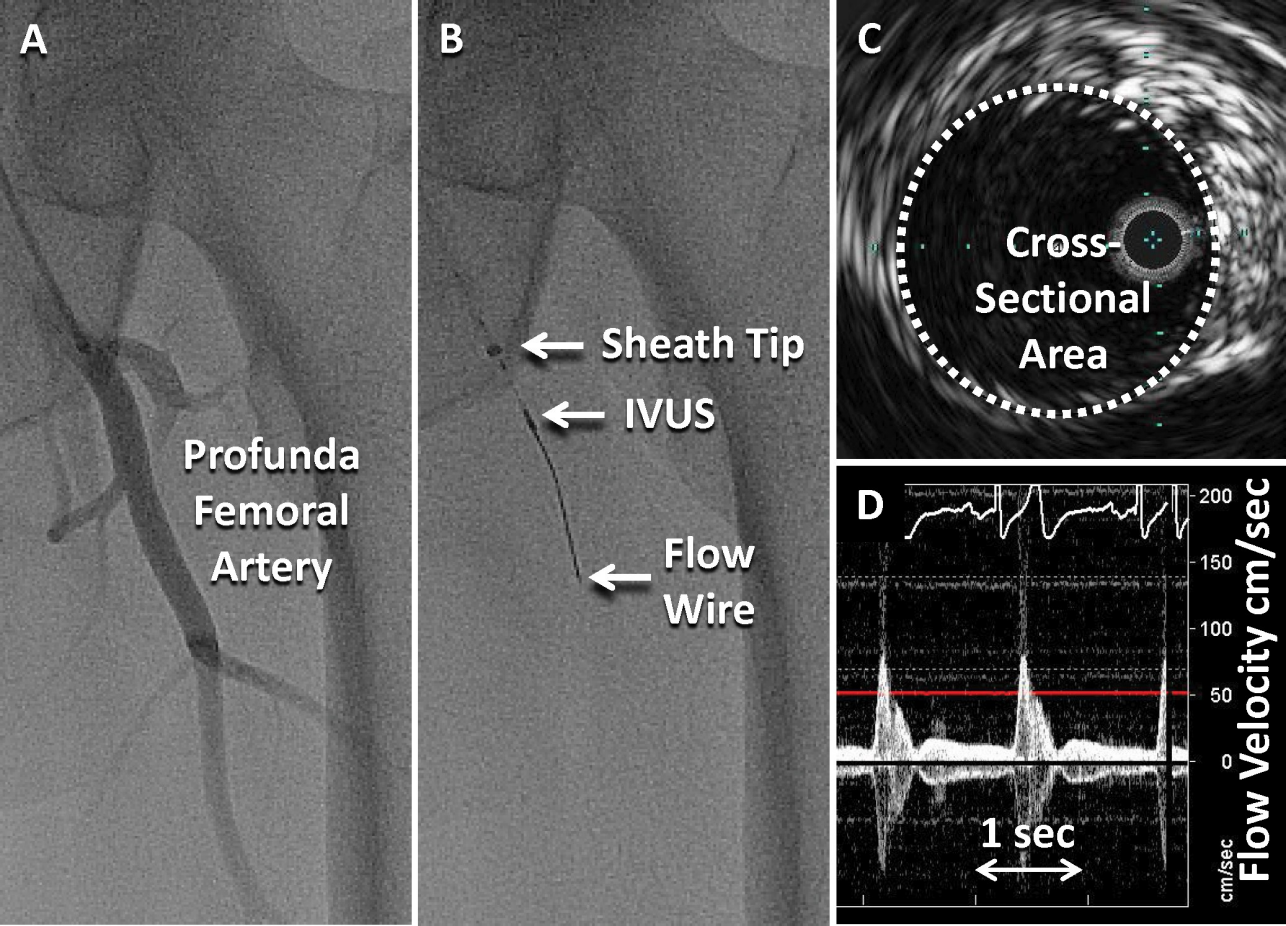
Catheter positions. A. Angiogram of the left profundal artery. B. Fluoroscopy showing the tip of the sheath, the intravascular ultrasound (IVUS) catheter, and the Doppler Flow wire. C. IVUS image showing the measurement of the cross-sectional area. D. Flow velocity integral used to estimate blood flow volume.
